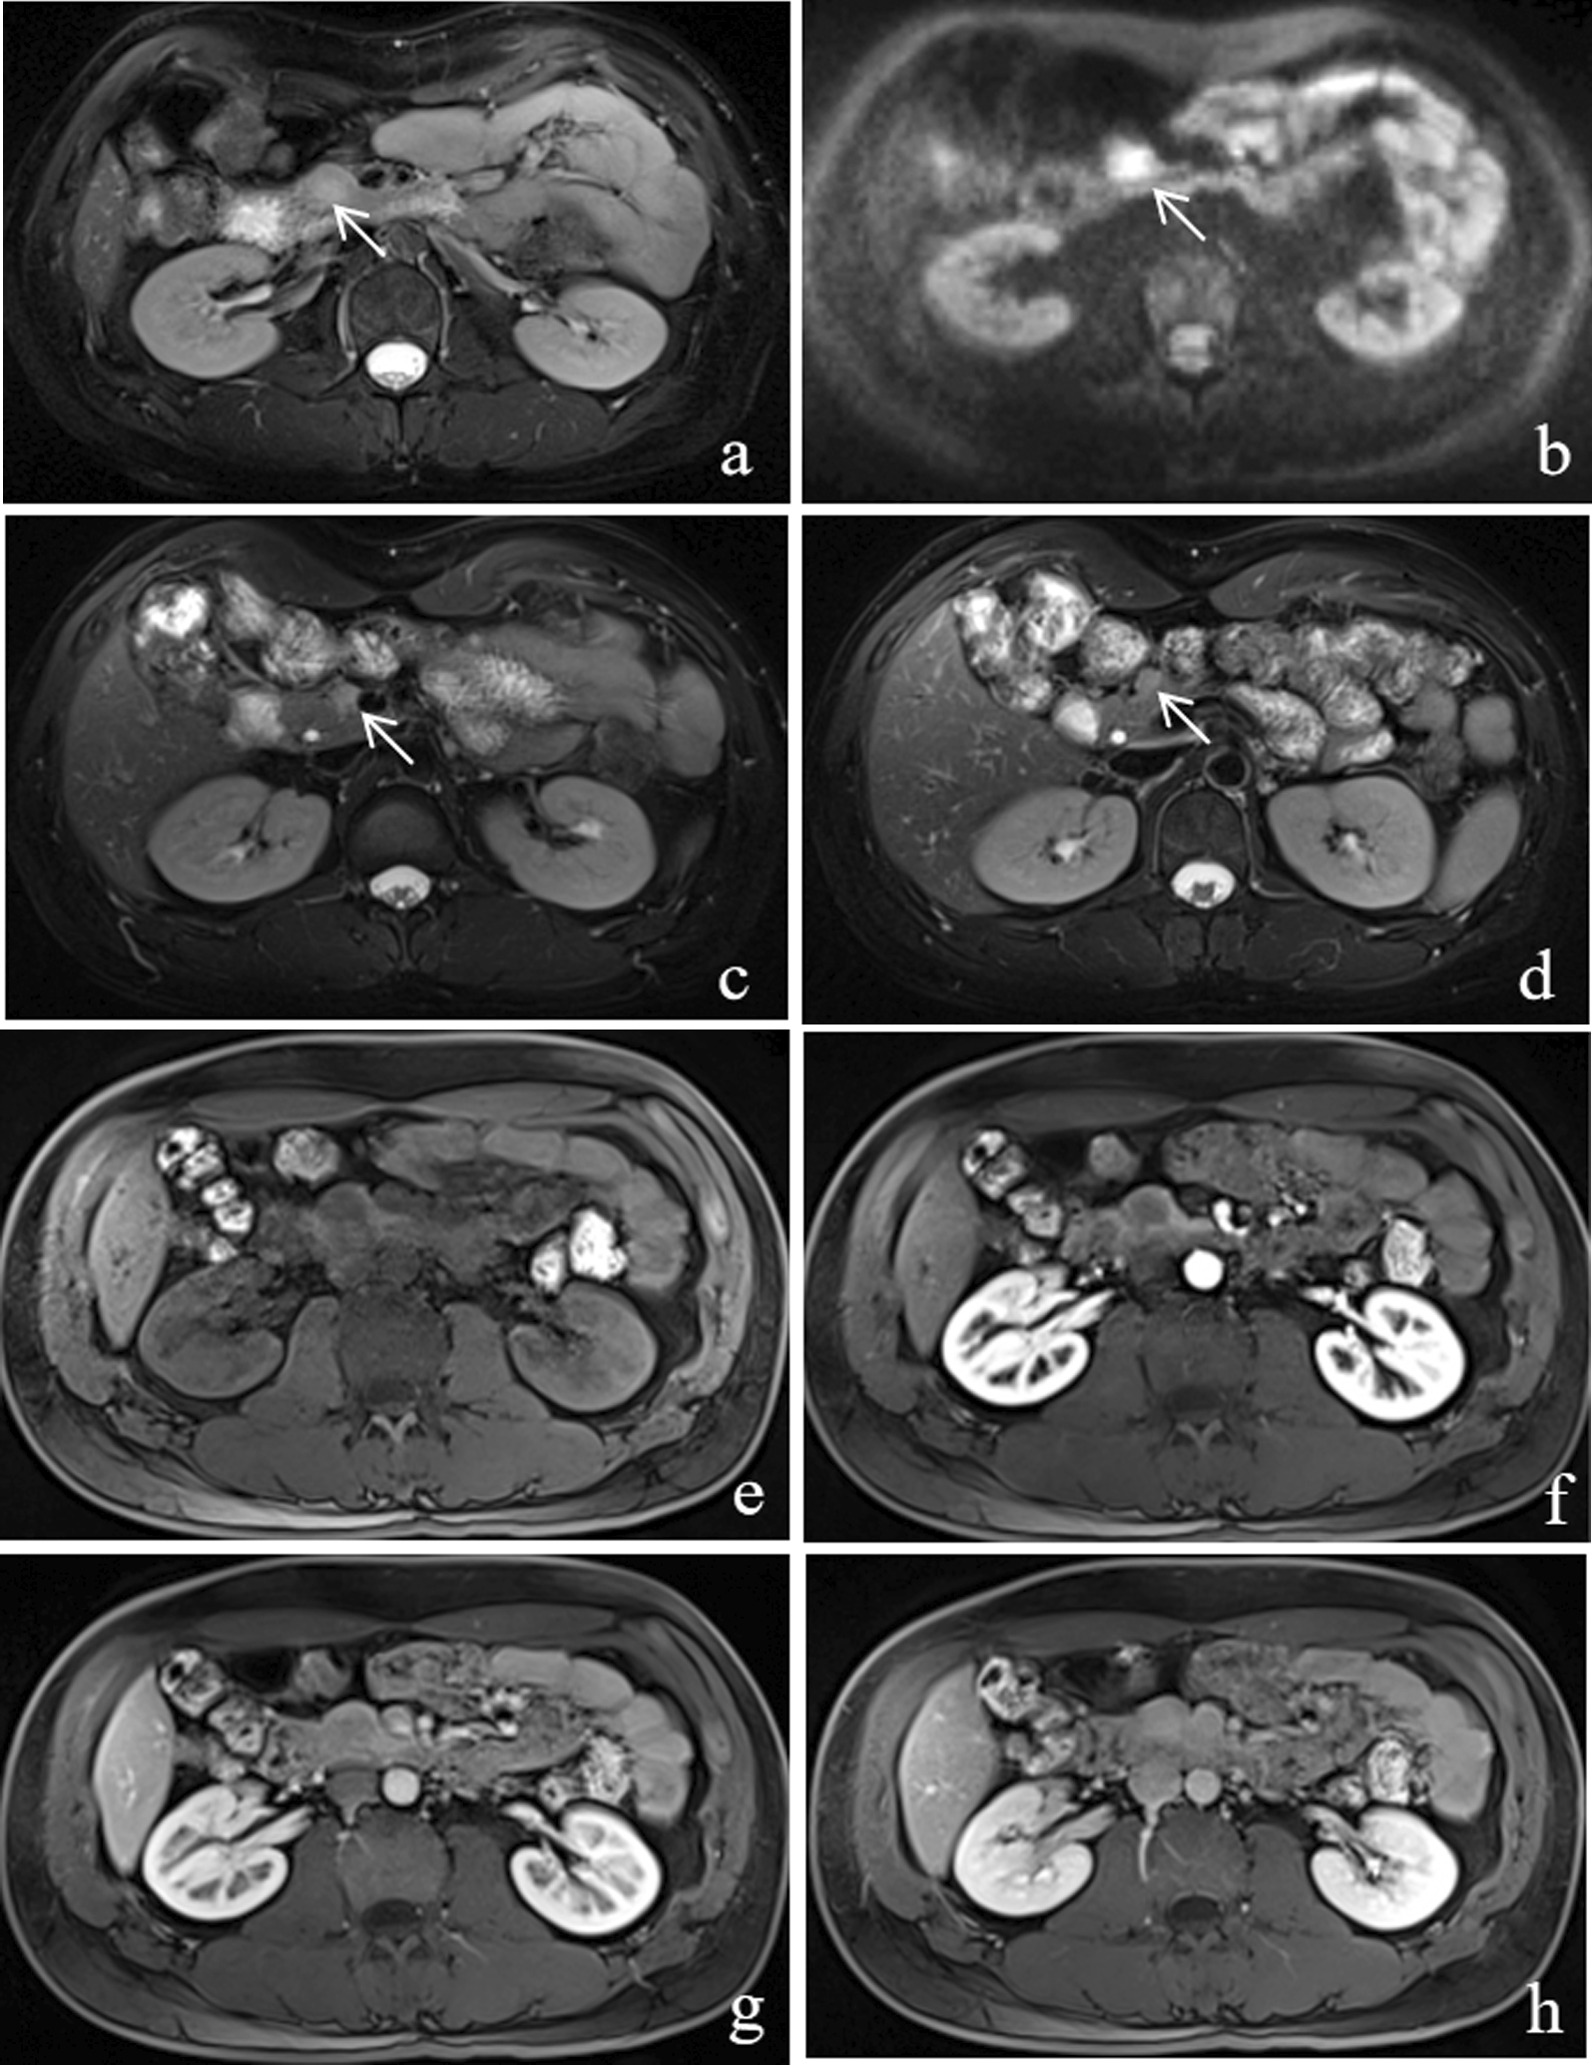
A 30-year-old woman had mass-forming AIP with multiple masses in the pancreas. a T2WI, a round-shaped mass located in the pancreatic head (arrow) is observed as hyperintense. b On DWI (b = 800 s/mm^2^), the mass appears hyperintense compared to the pancreatic parenchyma (arrow). Another two small, round-shaped, hyperintensity masses are located in the pancreatic head (c) and neck (d) (arrows). e Unenhanced T1-weighted MR image exhibits a round hypointense nodule in the head of the pancreas. f On the AP image, the mass demonstrates a well-defined, obvious hypointensity. g On the PP image, the mass shows subtle hypo- or isointensity. h The DP image demonstrates the homogeneous hyperintensity of the mass
